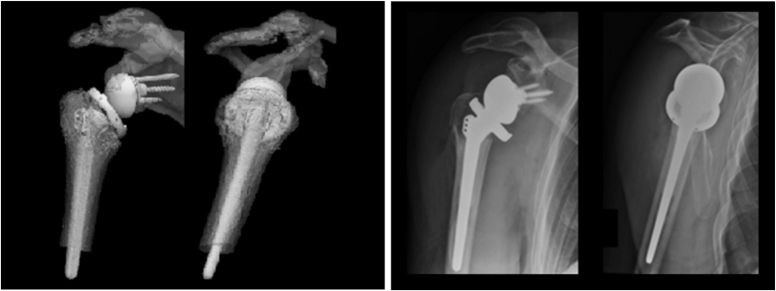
Postoperative AP and lateral views of the virtually planned a reverse shoulder arthroplasty (*left*) and postoperative radiographs (*right*), showing good position and size of the implant and anatomic reduction of the tuberosities. *AP*, anteroposterior.
